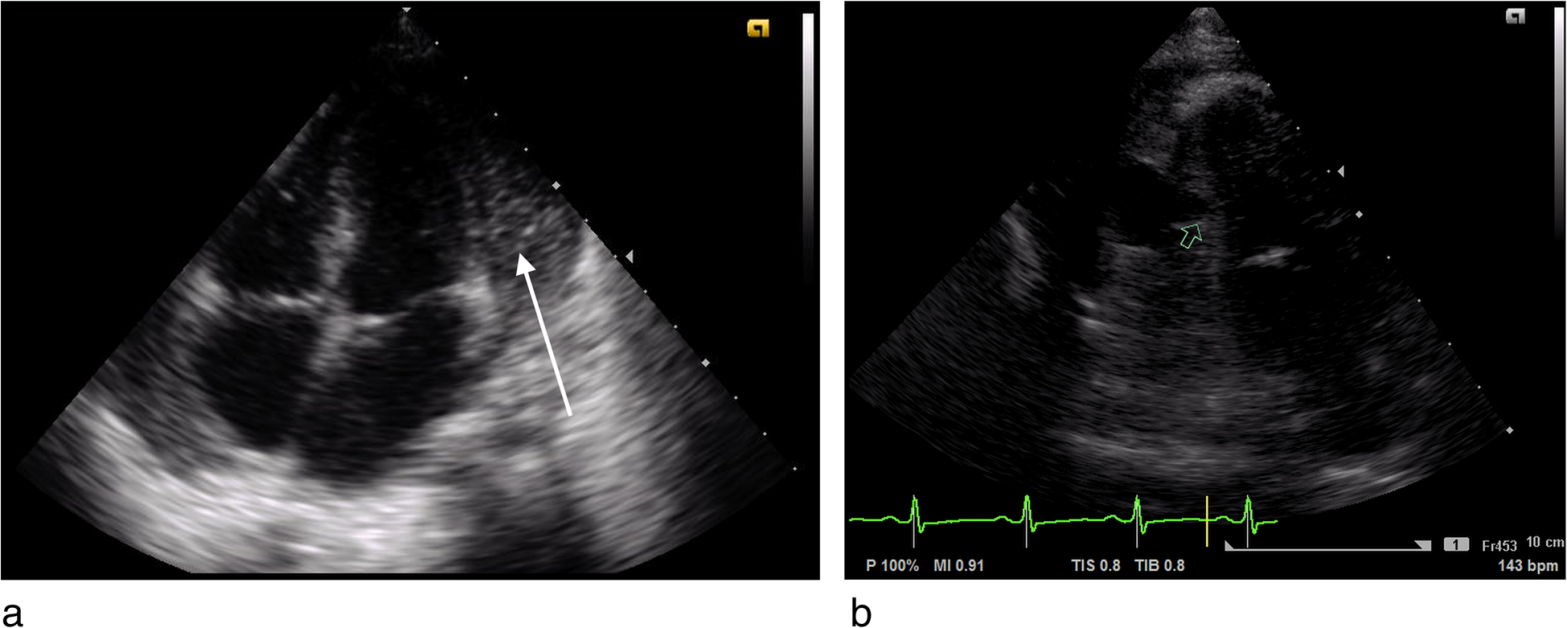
a Four chamber apical view, with organized purulent pericardial effusion 15–20 mm thick, with limited fluidity. b Apical 2 chamber view showing the left ventricle with an anechoic area (pseudo-aneurysm) lateral of the left ventricle free wall, with discontinuity of left ventricle wall, forming the communication between left ventricular inner space and the pericardial anechoic area
